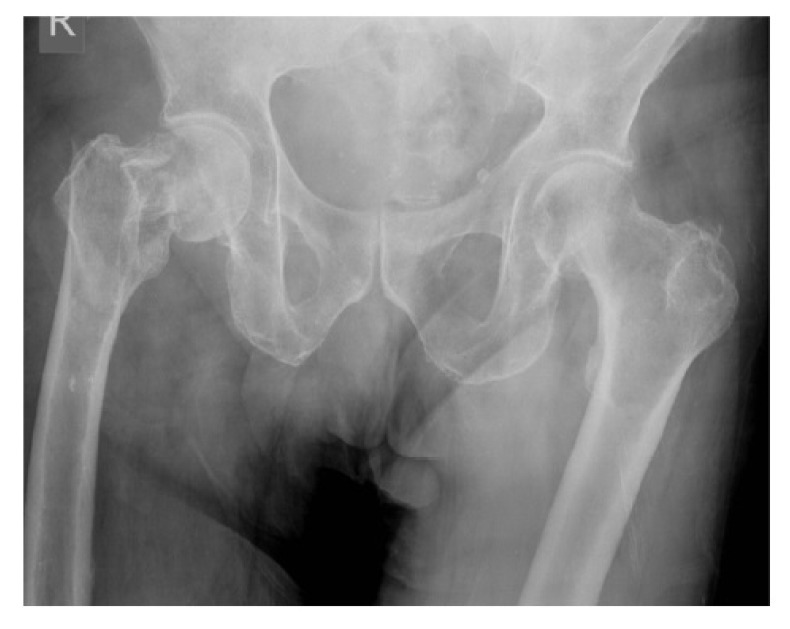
Anterio-posterior pelvic radiograph of Case 1 following the fall (a).
